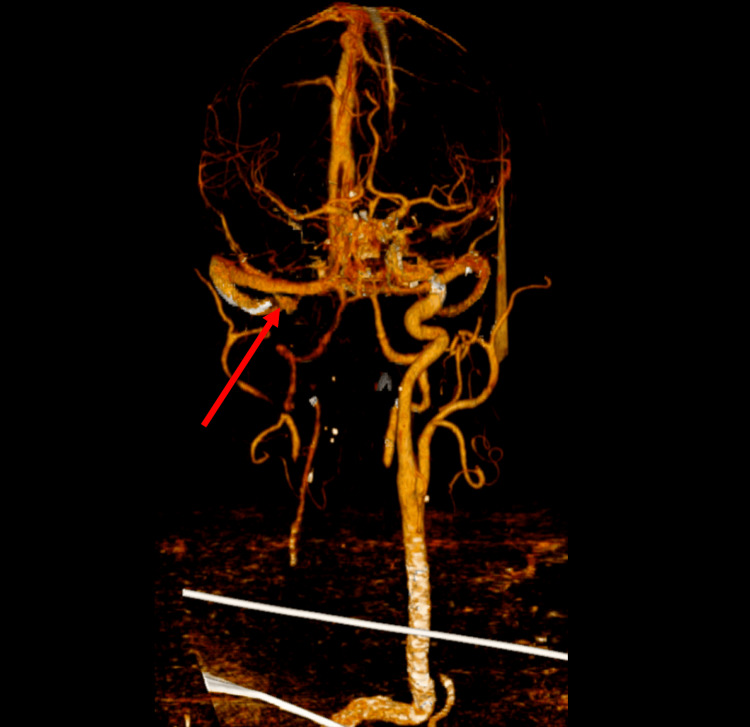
3D CT angiography reconstruction of the head and neck vessels demonstrating a right carotid artery occlusion alongside the arterial branches supplying the brain The occlusion is marked by the red arrow. 3D: three dimensional, CT: computed tomography
